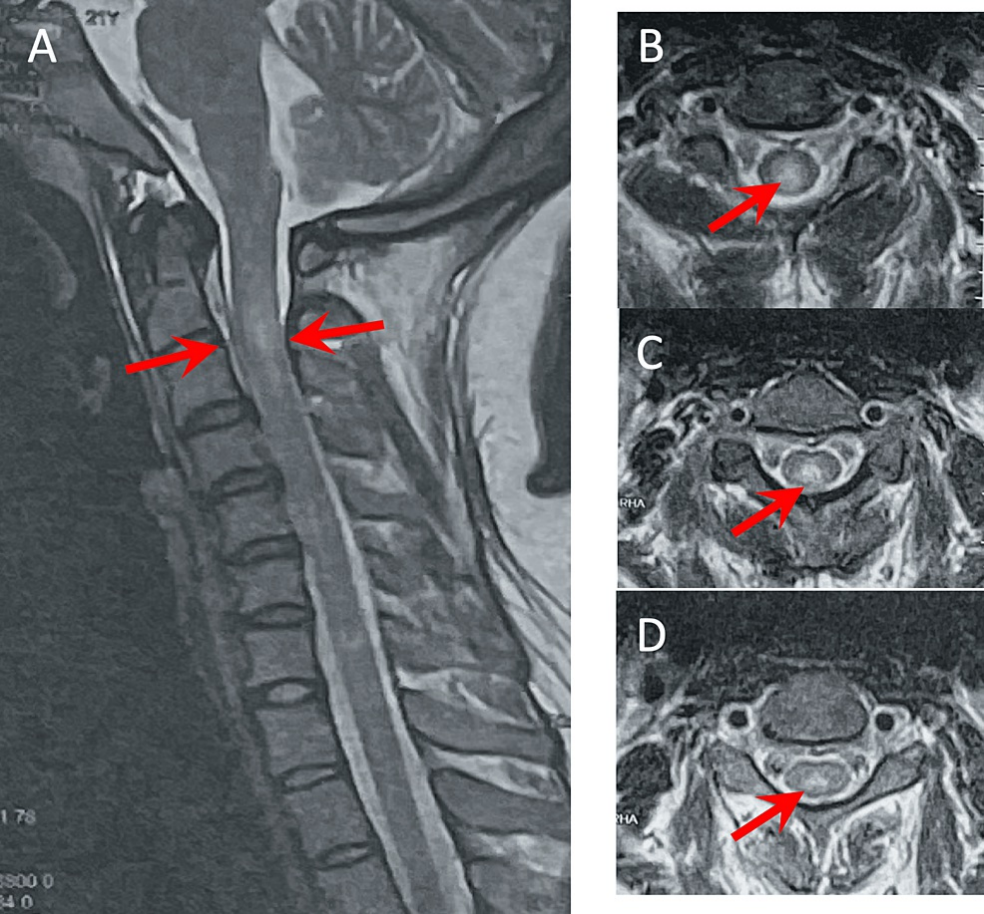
T2 weighted cervical MRI study showing focal 2.0 cm heterogeneously enhancing lesion (red arrows) involving the central intramedullary region at C2 to upper C3 levels, associated with subtle cord expansion and minimal adjacent cord edema A: T2 weighted cervicothoracic MRI sagittal cut B: T2 weighted cervical MRI transverse cut at upper C2 C: T2 weighted cervical MRI transverse cut at lower C2 D: T2 weighted cervical MRI transverse cut at upper C3
